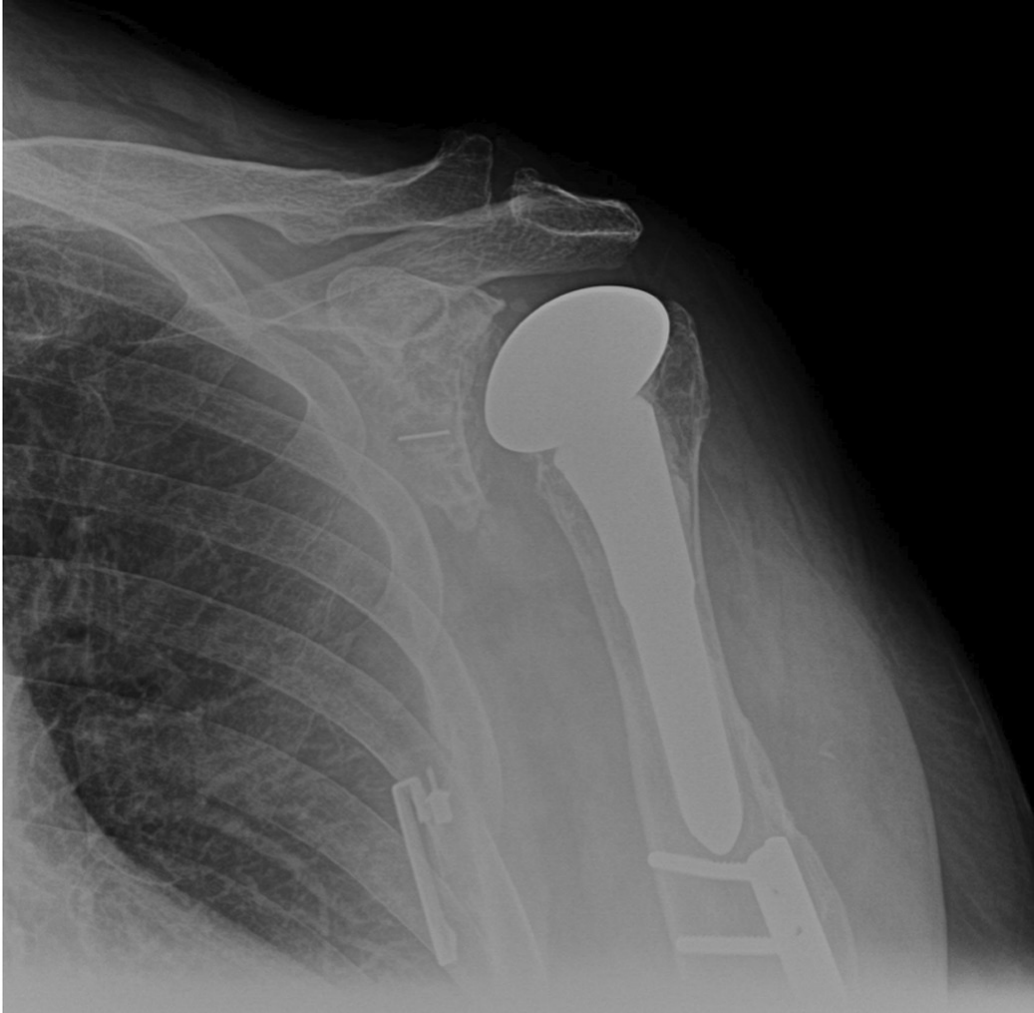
Postoperative radiographs of the left shoulder in anterior to posterior (AP) view depicting intact total shoulder arthroplasty hardware following arthroscopic rotator cuff repair. Intact humeral shaft ORIF without osseous complication is also seen.
